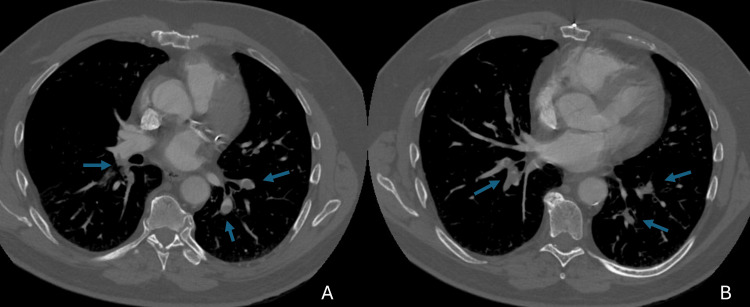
CT angiogram of chest (A, B) showing multiple bilateral pulmonary emboli (arrows)
